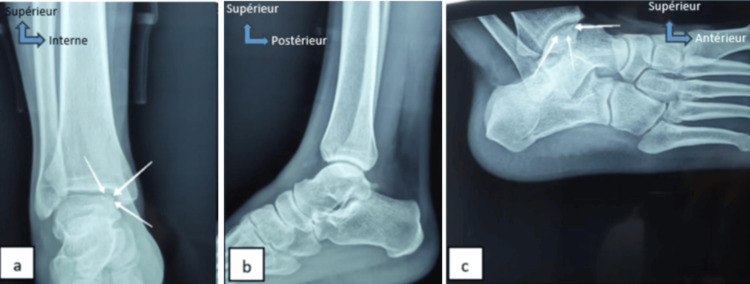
X-rays of the right ankle of case 1 (face (a), profile (b), 3/4 incidence (c)) shows osteochondral lesions of the medial part of the talus dome (white arrows).
